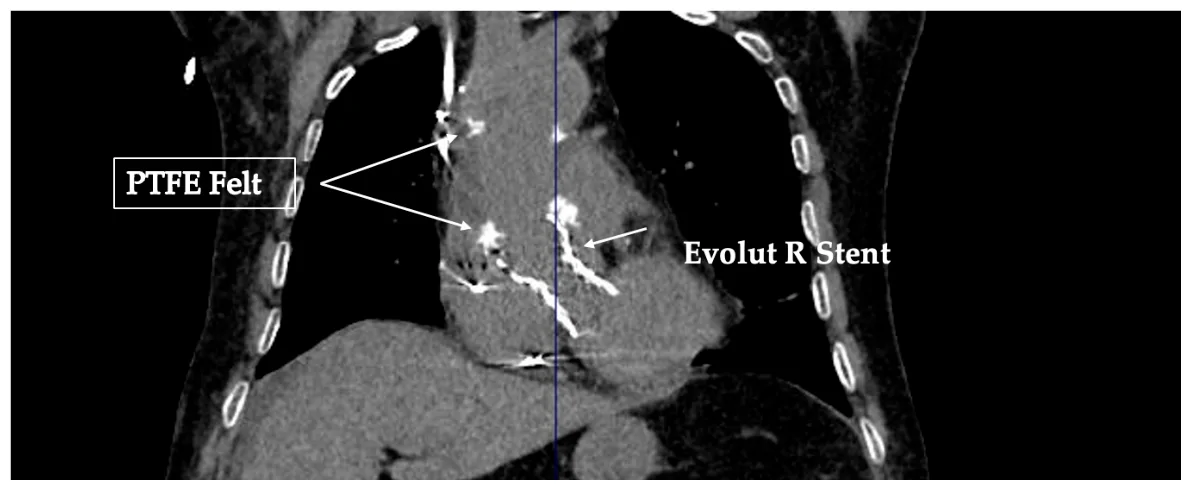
Postoperative CTA showing successful ascending aortic replacement with preservation of the previously implanted self-expanding TAVI prosthesis. Proximally, a Teflon felt strip is interposed between the transcatheter valve stent frame, the native aortic wall, and the vascular graft as part of the sandwich reinforcement technique. Distally, a second Teflon felt strip is positioned between the vascular graft and the native aortic wall, providing additional anastomotic support.
